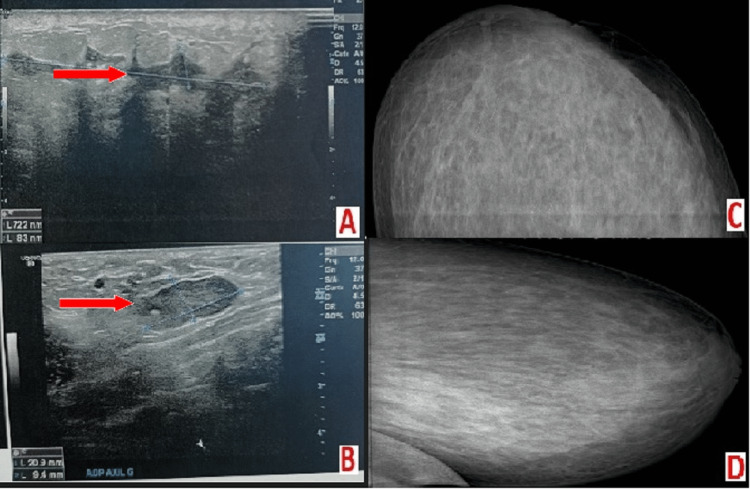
Ultrasound scans. Left breast showing widespread infiltration of the lower quadrants structure (A), associated with homolateral axillary adenopathy of uniform ultrasound ISO structure (B). Mammogram of the left breast, external face (C) and profile (D) showing an opaque veil heterogeneous and little restricted of the lower quadrants.
